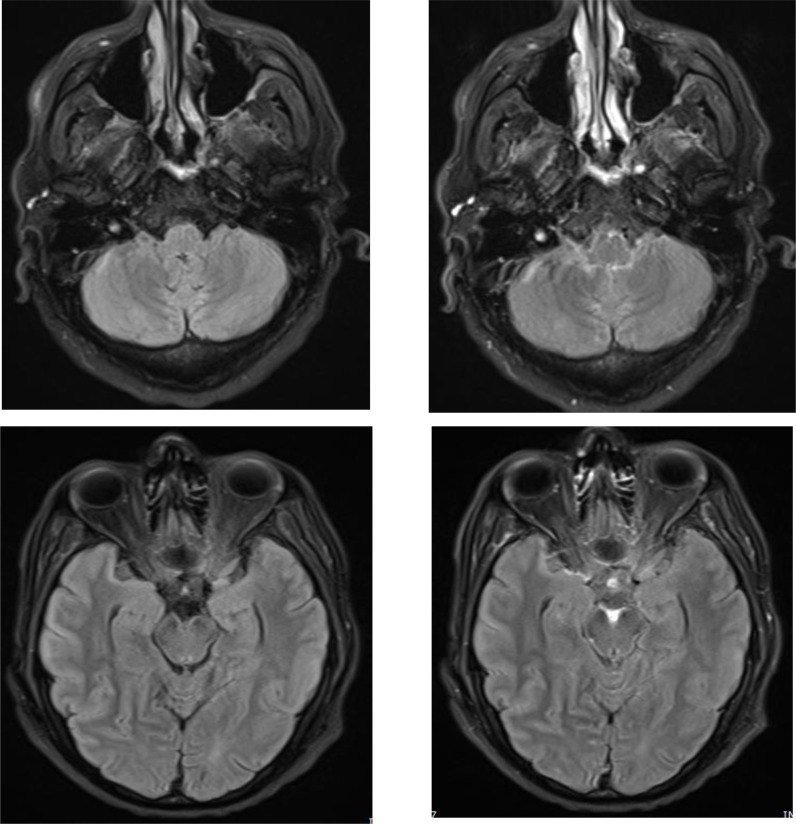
Axial FLAIR weighted images (WI) of the brain at the level of the posteriro fossa and temporal labes and after gadolinium based TV contrast injection showing basal meningeal thickening and enchancement predominantly circumscribing the brain stem structures and the interpeduncular cistern.
